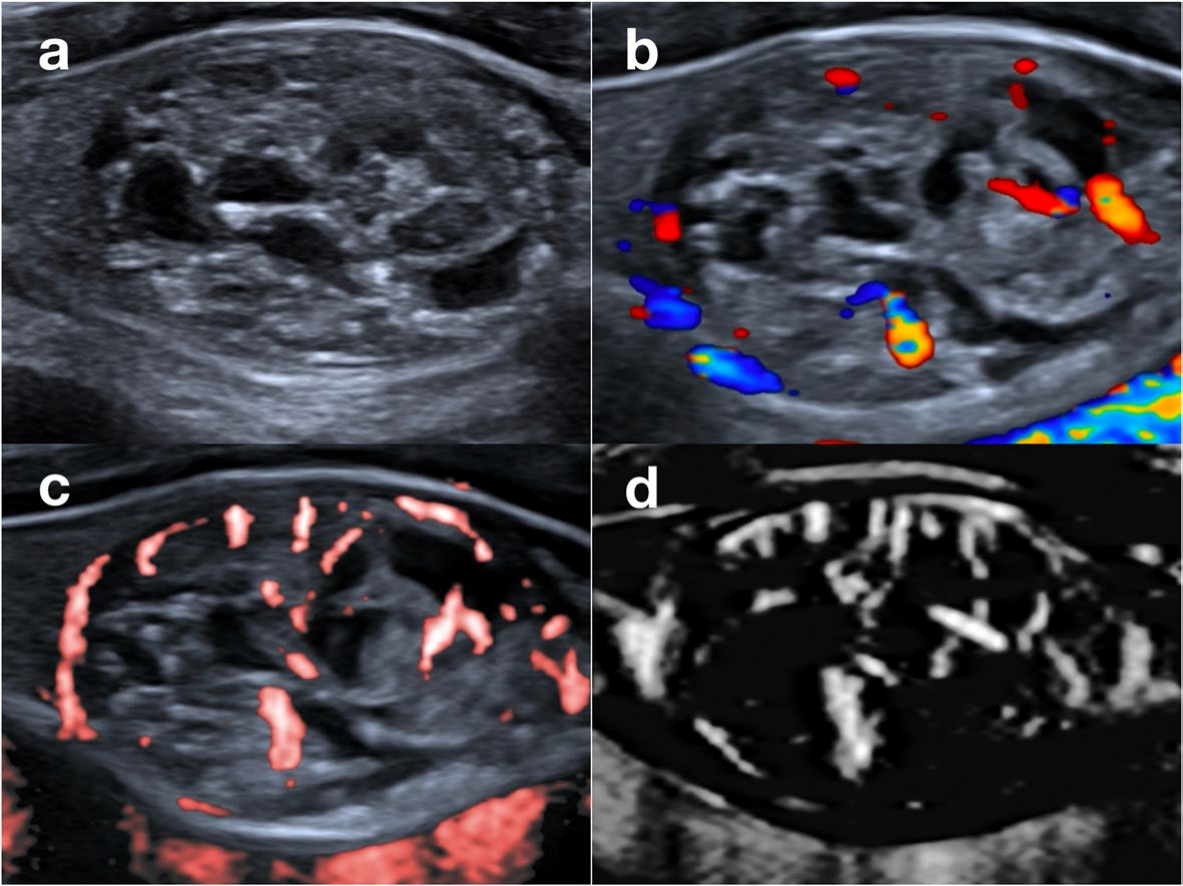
Ultrasound images of a nodular goiter in a 49 female patient. a The lesion presented as a mixed solid and cystic nodule on greyscale. b CDFI showed several dot- and rod-like flow signals inside and around the nodule. c CSMI showed a wheel-like pattern. d MSMI showed a wheel-like pattern
